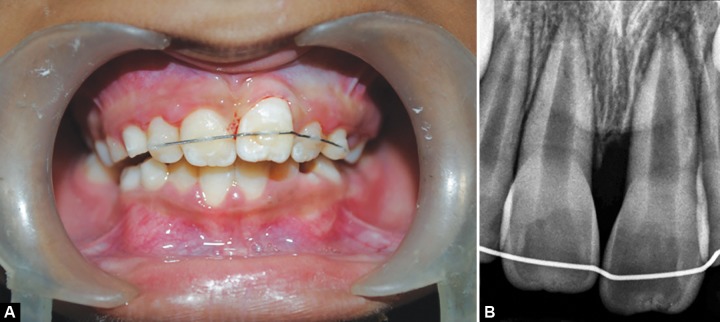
Immediate postoperative clinical picture and intraoral periapical digital radiograph showing splint
